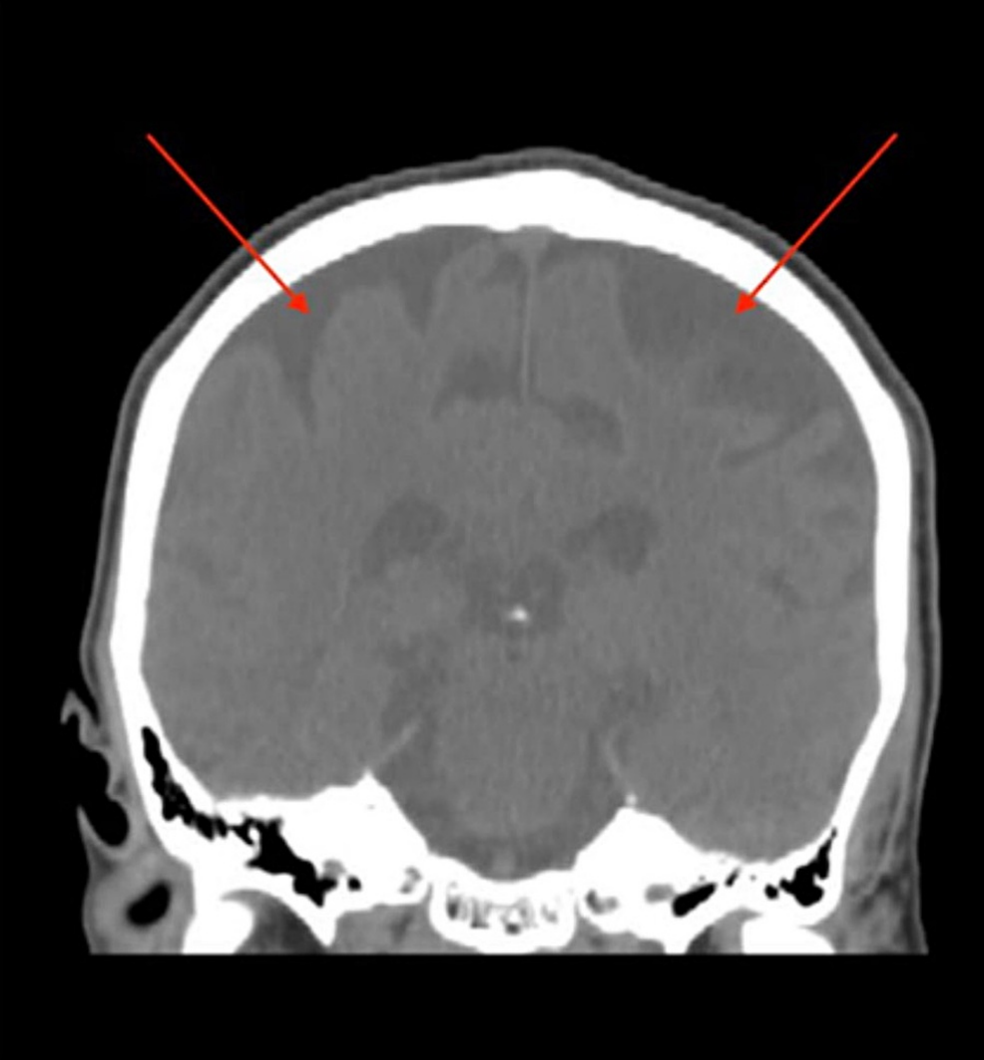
Coronal T1 diffusion-weighted head MRI, with hypoattenuation representing marked cortical atrophy (red arrows)
